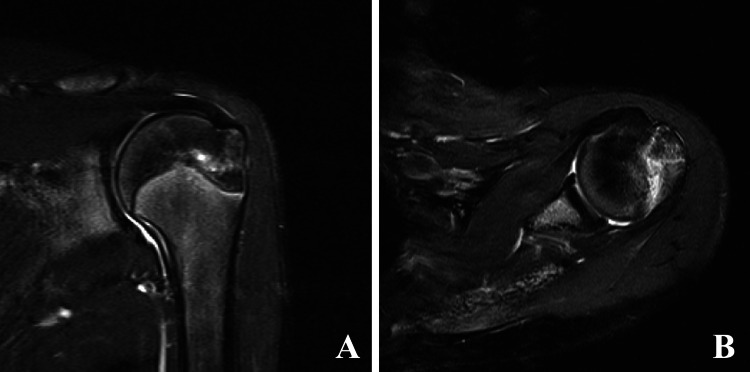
MRI of the left shoulder at six weeks post-injury. A, Coronary MRI STIR slice. B, Axial MRI STIR slice. MRI, magnetic resonance imaging; STIR, short-tau inversion recovery.
